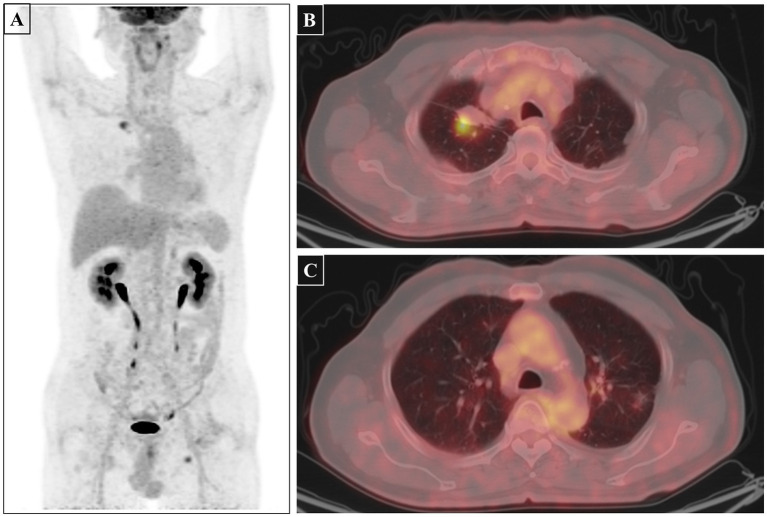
Examples of DeepSeek’s lung cancer staging errors (A:MIP), DeepSeek considered the upper lobe of the right lung (B:axial CT) to be the primary focus of lung cancer, but for the hypermetabolic nodule in the upper lobe of the left lung (C:axial CT), it gave two possibilities in the first two responses: primary lung cancer or intrapulmonary metastatic spread, and in the third time DeepSeek considered this nodule to be an intrapulmonary metastasis thus leading to a change in the M staging from M0 to M1a leading to an increase in the staging from IB to IVA.
